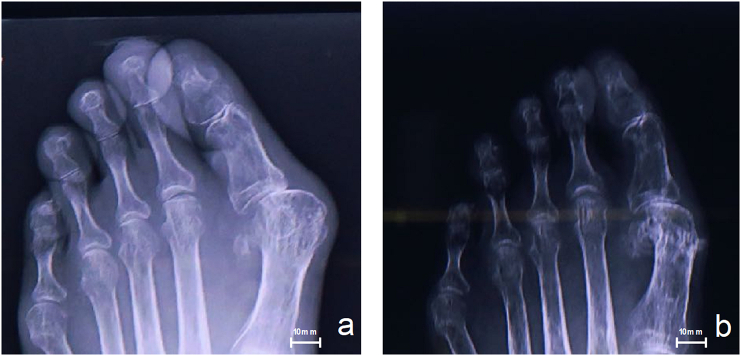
X-ray (a: pre operation, b: after three years of operation).
